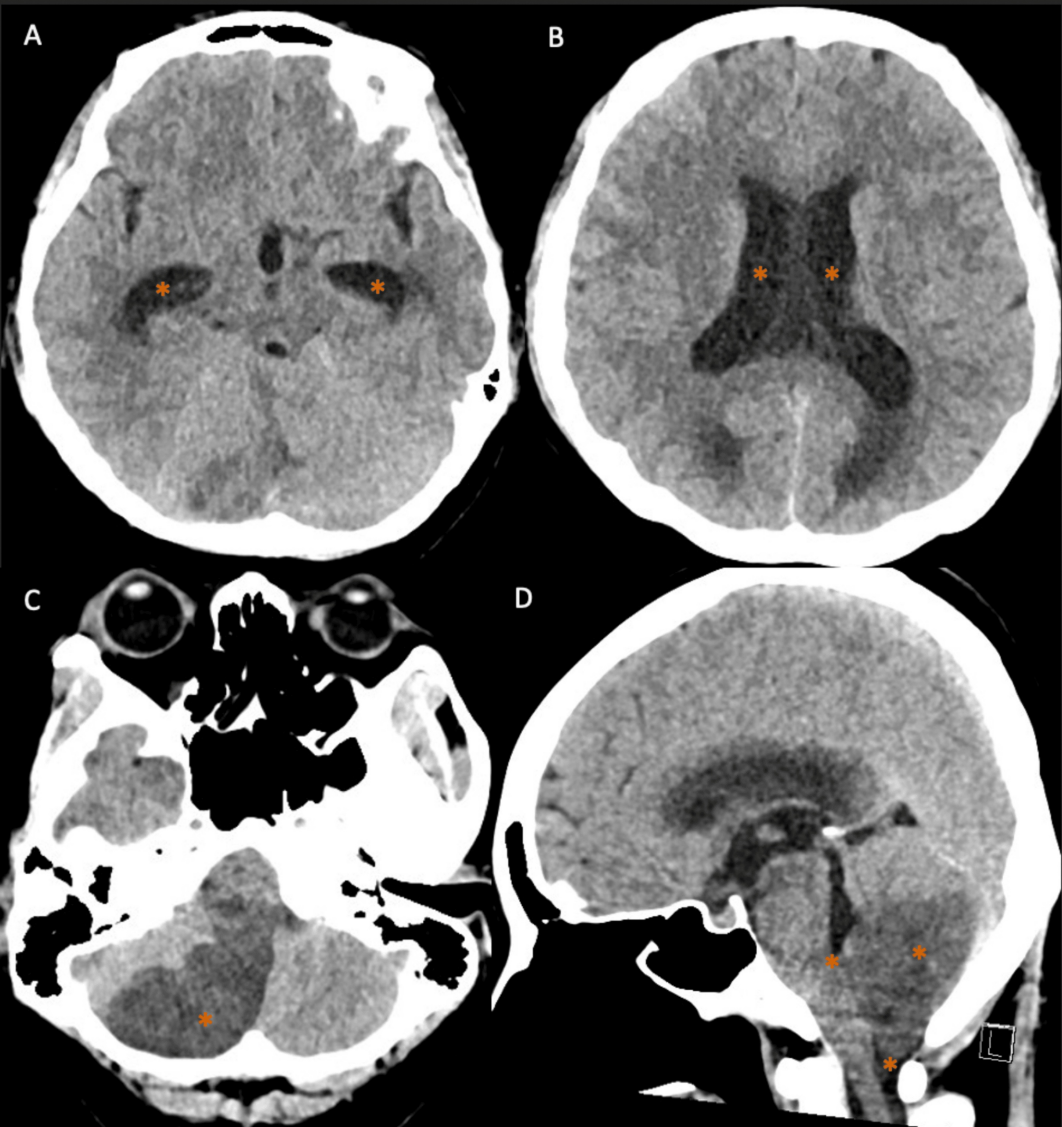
Follow-up CT of the brain following neurological deterioration (A-D) Well-established right cerebellar infarct in the posterior inferior cerebellar artery (PICA) territory, with associated edema and evolving obstructive hydrocephalus secondary to compression of the fourth ventricle and brainstem, mild crowding at the foramen magnum, and tonsillar descent measuring approximately 5 mm (orange asterisks).
